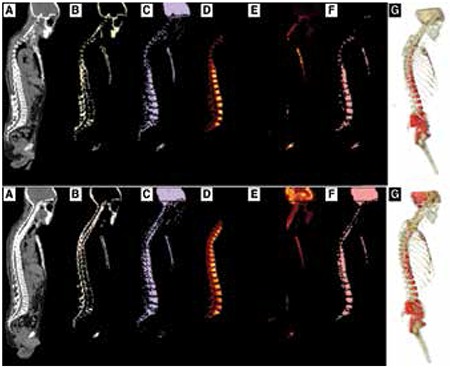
Full body images at various steps of the BM extraction process with the top row showing 18F-FLT and bottom row showing 18F-FDG results. (A) CT image (B) Extracted cortical bone using probabilistic LDA (C) Extracted trabecular bone following probabilistic LDA (D) Activity in trabecular bone in thoracic and lumbar vertebrae regions (E) Activity in trabecular bone in the rest of the body excluding thoracic and lumbar vertebrae regions (F) Thresholded BM map generated after applying a statistical thresholding on PET image. (G) 3D rendering of BM in the entire body, with red regions showing red BM and yellow regions corresponding to yellow BM. The cortical bone overlay is showing using a transparent white overlay
